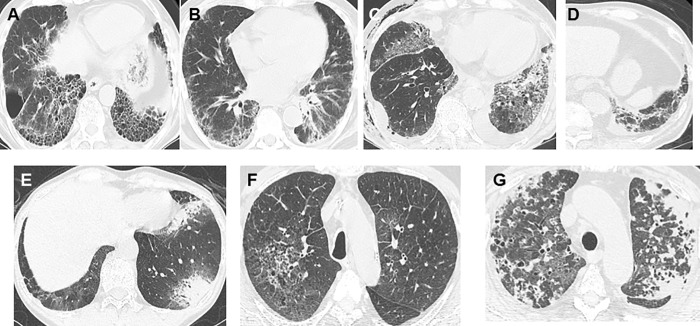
Pre-existing interstitial lung disease (ILD) showing subpleural distribution, honeycomb cysts, and bronchiectasis (usual interstitial pneumonia [UIP] pattern) (Fig 3A). Pre-existing ILD showing patchy ground-glass opacity with reticulation, traction bronchiectasis and bronchovascular bundle thickening (non-UIP pattern) (Fig 3B). Drug-related pneumonitis showing new diffuse ground-glass opacities, consolidation and traction bronchiectasis as well as pleural effusion, indicative of AIP pattern (Fig 3C). Drug-related pneumonitis showing new ground-glass opacities and bronchovascular bundle thickening, indicative of on-specific interstitial pneumonia (NSIP) pattern (Fig 3D). Drug-related pneumonitis showing new ground-glass opacities and consolidations with multifocal distribution, indicative of OP pattern (Fig 3E). Drug-related pneumonitis showing new diffuse faint ground glass opacities, indicative of HP pattern (Fig 3F). Drug-related pneumonitis showing diffuse new ground-glass opacities. Since the CT showed multiple consolidation (lung cancer) in both lungs, we could not classify the type of drug-related pneumonitis (unclassifiable pneumonitis) (Fig 3G).
